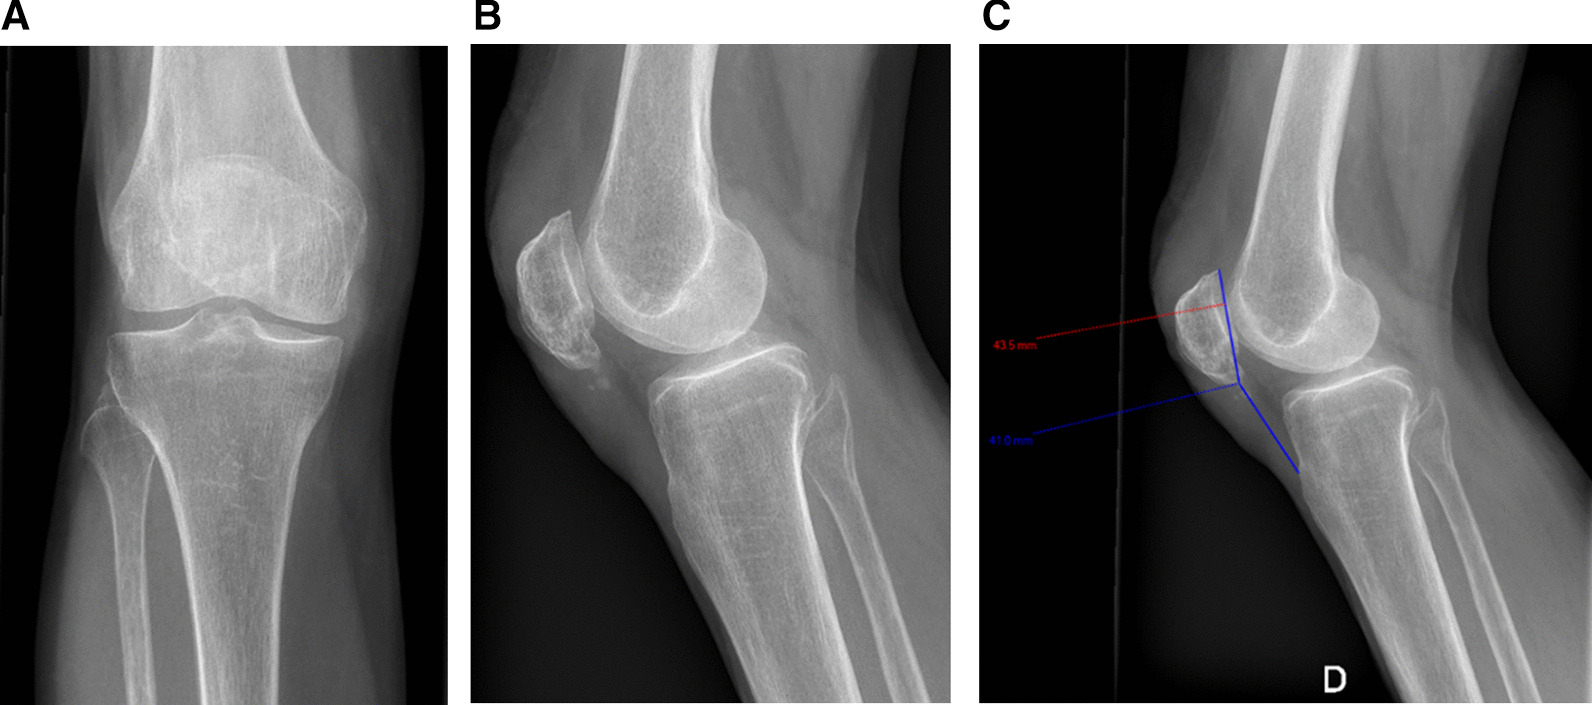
a, b, c: Month 6 post operative control x-ray—Insall Salvati ratio = 0,94
